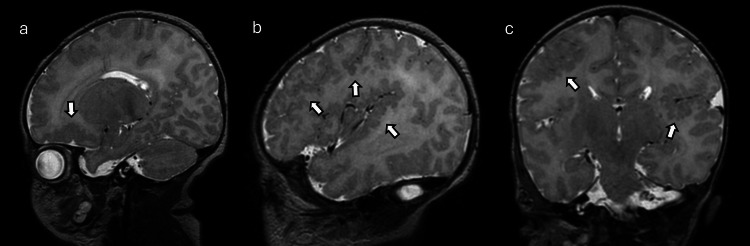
Cabezas X-linked intellectual disability in a nine-day-old boy with feeding difficulty (a), (b) sagittal, and (c) coronal T2W MRI showing extensive polymicrogyria involving bilateral perisylvian cortex and bilateral frontal lobes (arrows). DWI and ADC maps were normal. Images obtained by the authors from the institutional PACS. All images are de-identified and used in accordance with institutional policy. T2W: T2-weighted; DWI: diffusion-weighted imaging; ADC: apparent diffusion coefficient; PACS: picture archiving and communication system
